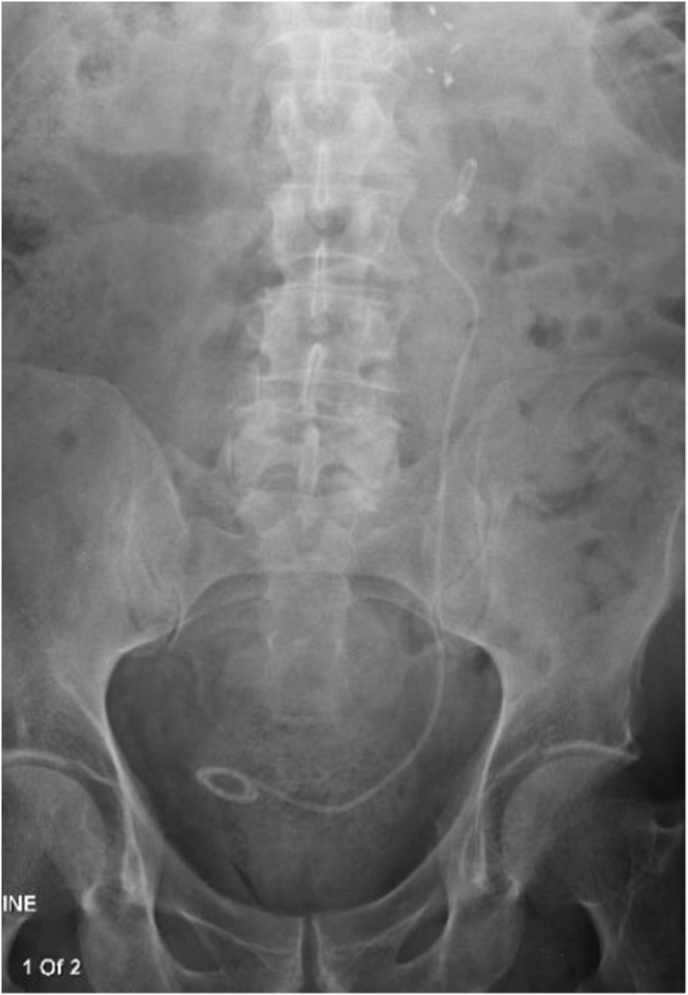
Left knotted ureteric stent on abdominal x-ray.
